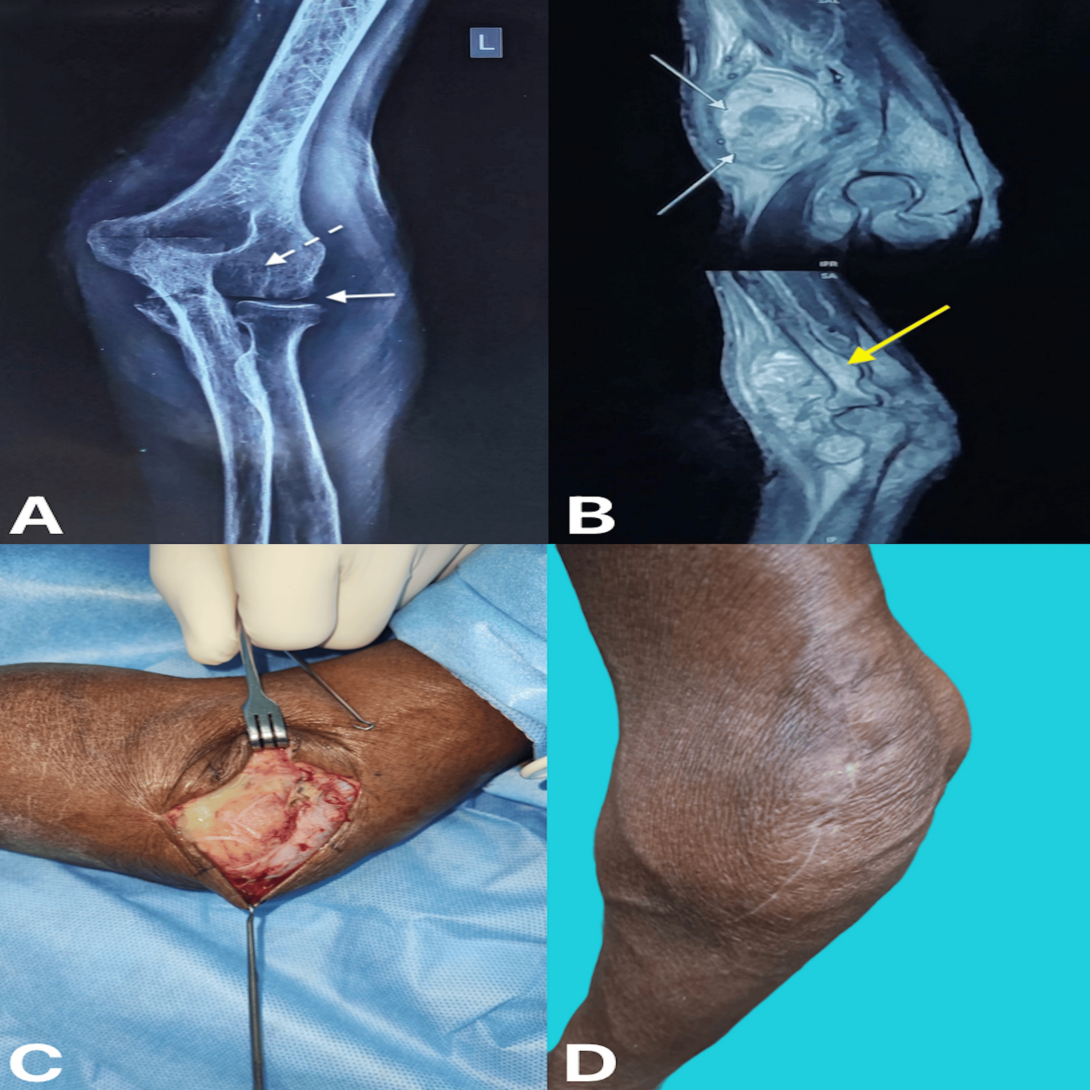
(A) Radiograph of anterior-posterior view of left elbow joint, (B) magnetic resonance imaging of left elbow showing sagittal section, (C) intra-operative picture showing infected synovium, and (D) four months post-operative clinical picture of left elbow (A) The bold arrow shows decreased joint space. The dotted arrow shows periarticular osteopenia. (B) The white arrow in the above image shows a soft tissue abscess. The yellow arrow in the image shows marrow edema.
